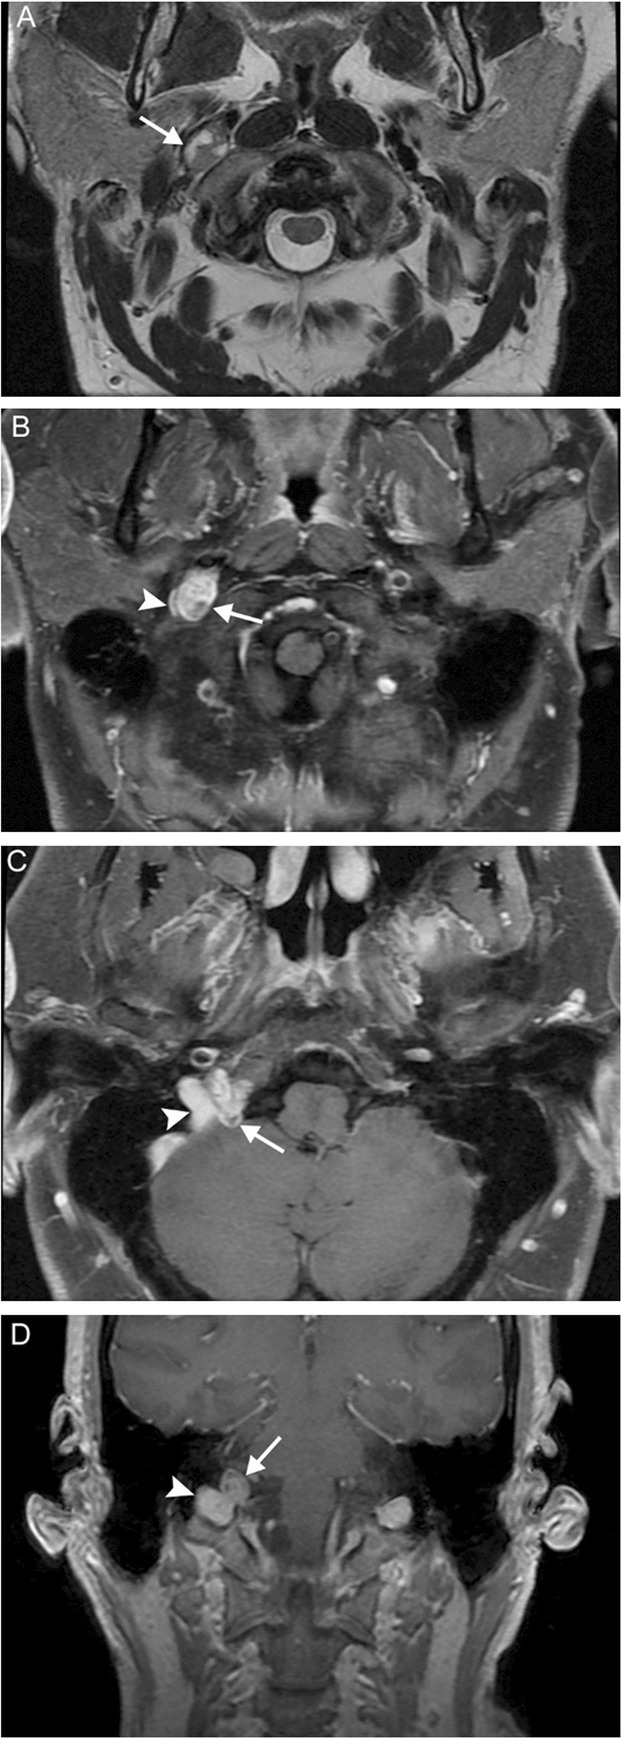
Vagal Schwannoma. A 53-year-old man with months of cough and hoarseness, thought to be reflux but not improved with proton pump inhibitor. Axial T2 (a), axial post-contrast T1 (b, c), and coronal post-contrast T1 (d) MR images reveal a well-circumscribed enhancing mass (arrows) with cystic components extending through the right jugular foramen (note the 'waist') into the carotid space. The right internal jugular vein (arrowheads) is compressed within the carotid sheath. Findings are characteristic for a vagal schwannoma, with intracranial and extracranial extent
